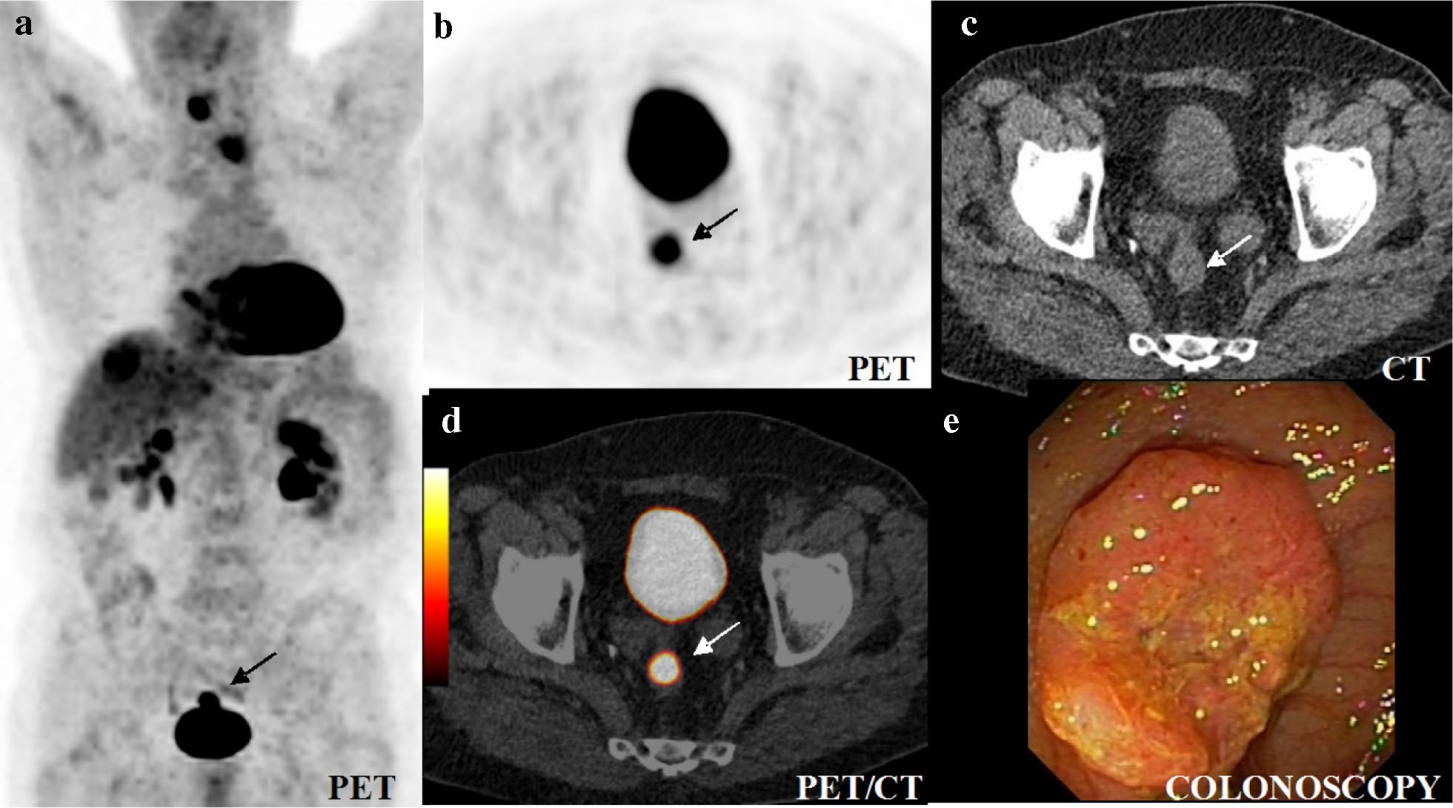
Colorectal cancer (*arrow*) as an incidental finding in the PET/CT restaging of oesophageal cancer with new lymph node and hepatic metastases. Maximum intensity projection (MIP) of PET captured in the coronal projection of the 360°-rotation (a), axial slices of PET (b), CT (c) and PET/CT (d) and colonoscopy (e). Note that the ^18^F-FDG-filled bladder can obscure carcinomas on the coronal maximum intensity projection (MIP) (a). Rotating MIPs or scrolling through axial PET slices (b) is mandatory to detect such carcinomas. The colorectal cancer, here with an SUV_max_ of 9.7, is clearly depicted on the axial PET images (b) in contrast to CT (c)
